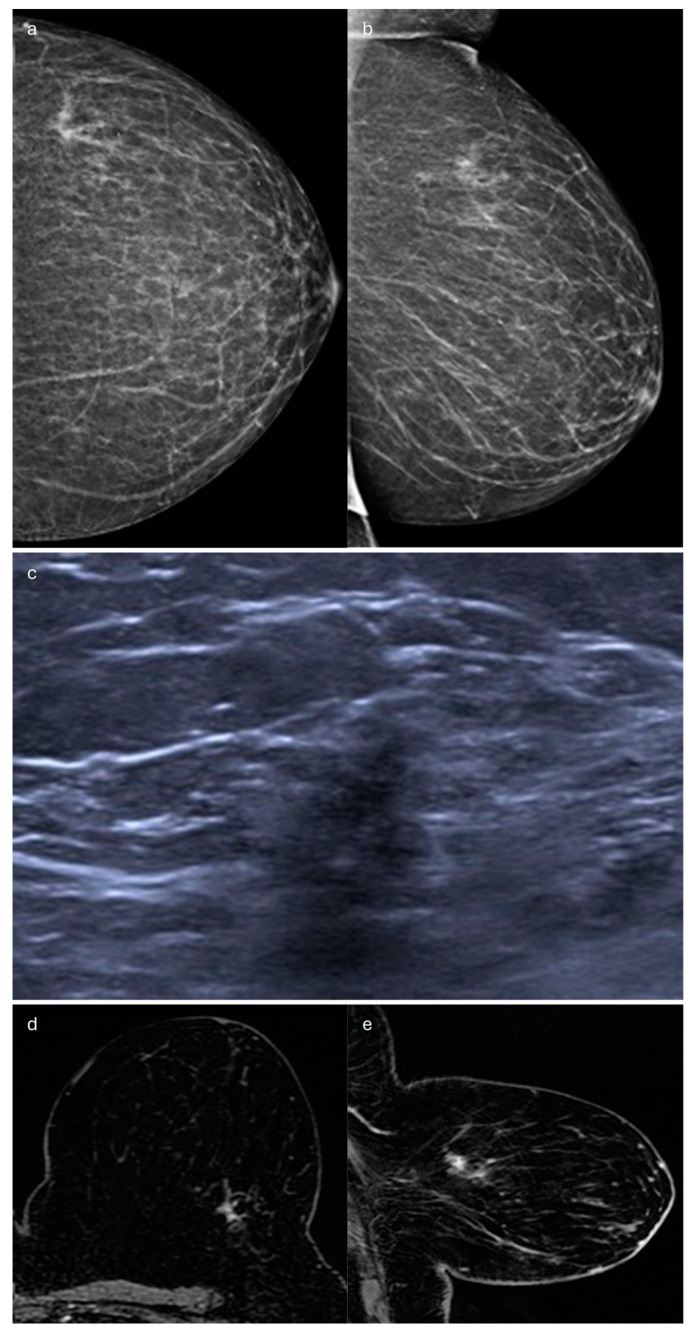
Invasive lobular carcinoma in a 53-year-old patient. (a) Cranio-caudal and (b) medio-lateral oblique mammograms of the left breast show an architectural distortion in the upper-outer quadrant, (c) corresponding to an irregular-shaped hypoechoic lesion with spiculated margins on ultrasound. (d) Axial and (e) sagittal T1-weighted fat-suppressed dynamic contrast-enhanced MRI images reveal an irregular mass with spiculated margins in the upper-outer quadrant.
